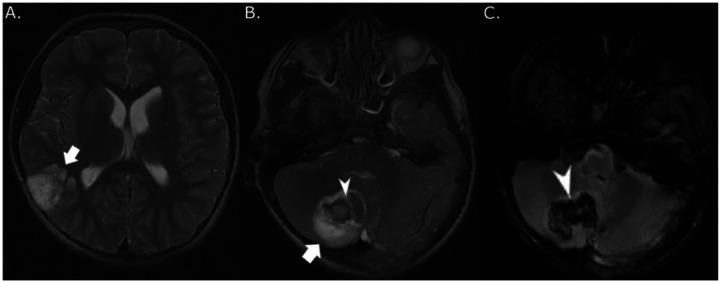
Radiologic characteristics of a multifocal DNET. (A) Axial T_2_-weighted MRI in patient 14 showed hyperintense, septated lesion in right temporal lobe (white arrow), which was confirmed to be a dysembryoplastic neuroepithelial tumor (WHO grade 1). The deeper aspect of the tumor was shown to have a hemorrhagic area by gradient echo sequence. (B) Axial T_2_-weighted MRI in patient 14 obtained on the same day showed a separate lesion in the right cerebellum (white arrow) with hyperintense rim and an eccentric isointense central area (white arrowhead) compatible with hemorrhage. This lesion was also confirmed to be a dysembryoplastic neuroepithelial tumor (WHO grade 1). (C) Gradient echo sequence confirmed cerebellar tumor hemorrhage (white arrowhead).
